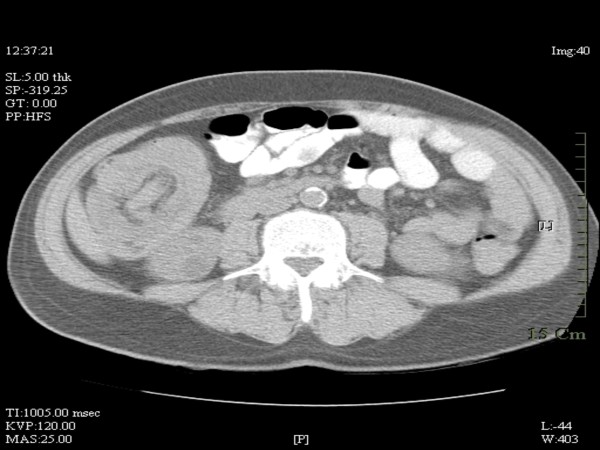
CT scan of abdomen showing ileo-colic intussusception.
